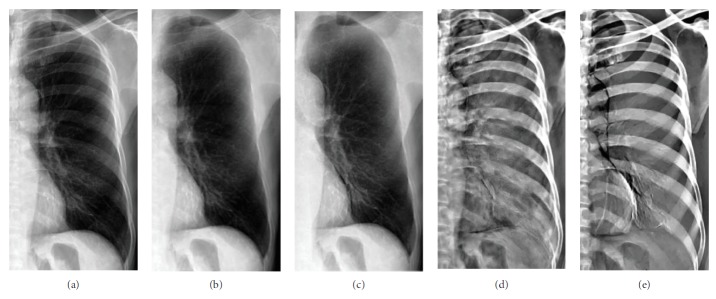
Decomposition results of a chest radiograph with motion artifacts: (a) the left lung field of a chest radiograph; (b) the reconstructed soft tissue image by use of our method; (c) the actual DES soft tissue image; (d) the reconstructed bone image by use of our method; (e) the actual DES bone image with obvious motion artifacts.
